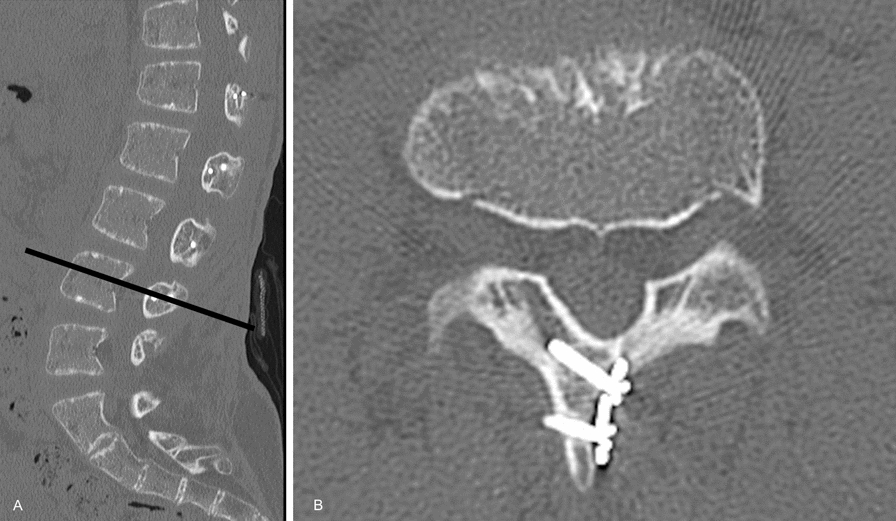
At the 2-year postoperative follow-up, computed tomography images confirmed proper bony fusion at the plate fixation sites (A: sagittal plane, B: transverse plane)
